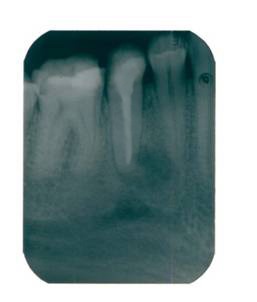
Radiograph after conventional endodontic treatment
